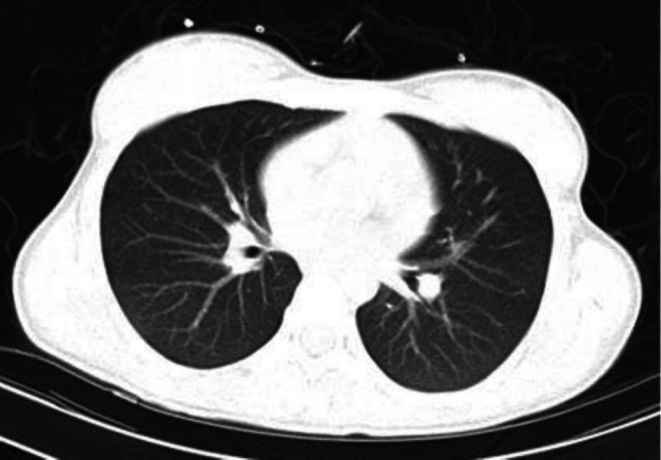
Computed tomography of the patient was negative for pulmonary sarcoidosis.
